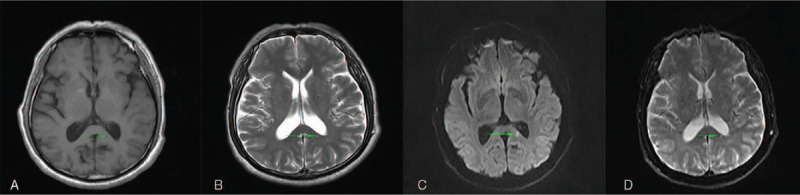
Brain magnetic resonance imaging after a 6-mo follow-up. Brain magnetic resonance imaging showed an isolated lesion in the splenium of the corpus callosum with hypointensity on T1-weighted imaging (A) and the apparent diffusion coefficient map (D) and hyperintensity on T2-weighted imaging (B), diffusion-weighted imaging (C).
